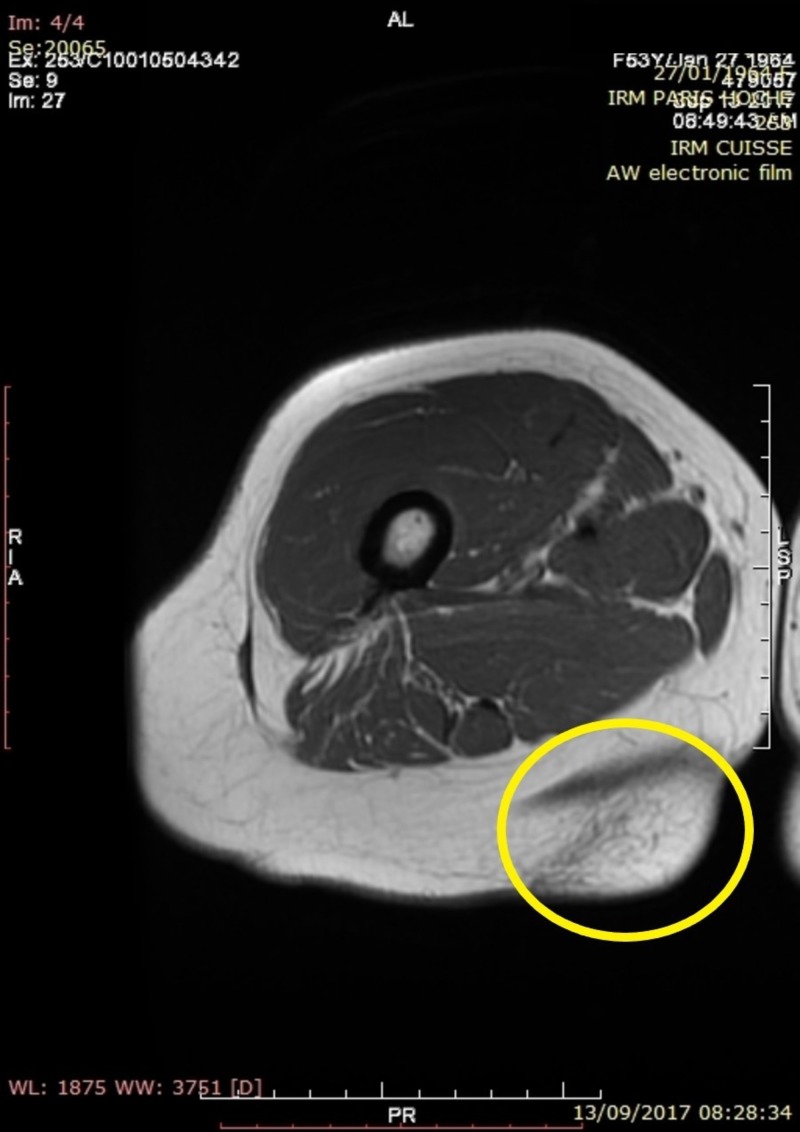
Axial view of a lower extremity MRI showing the pseudolipoma marked by the yellow circle. MRI: Magnetic resonance imaging
